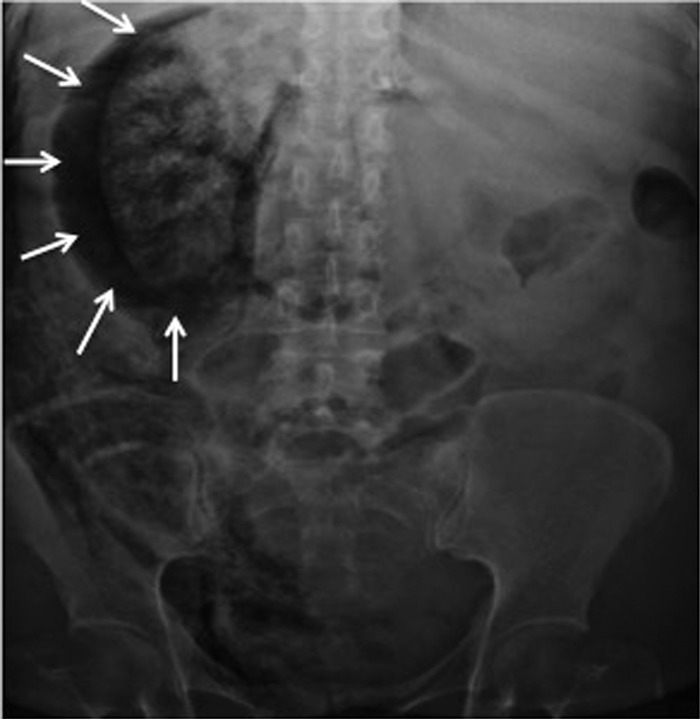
Abdominal x-ray shows gas distributed in the topography of the right kidney (arrows).
